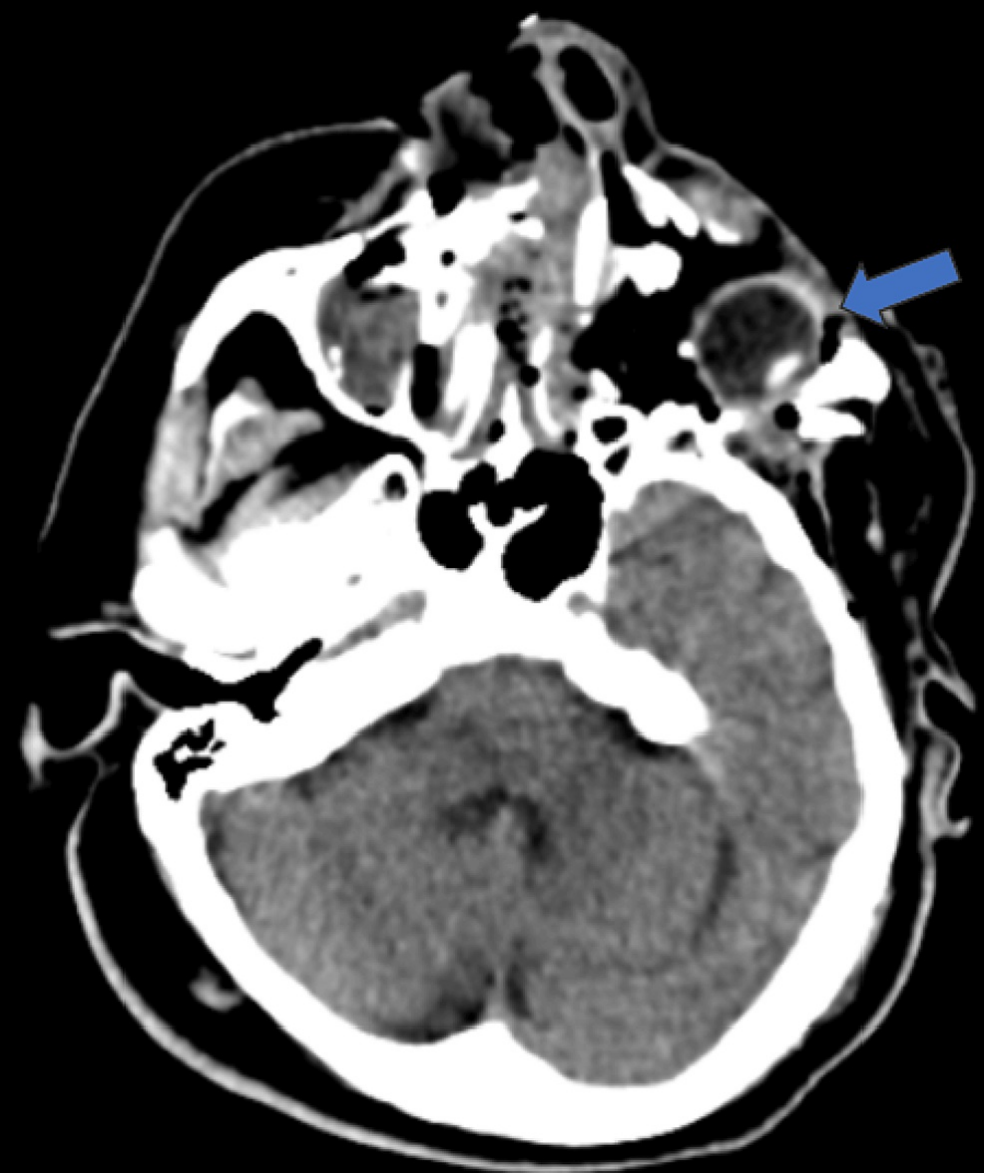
CT scan showing left globe luxation inferotemporally (blue arrow). The globe was intact.
